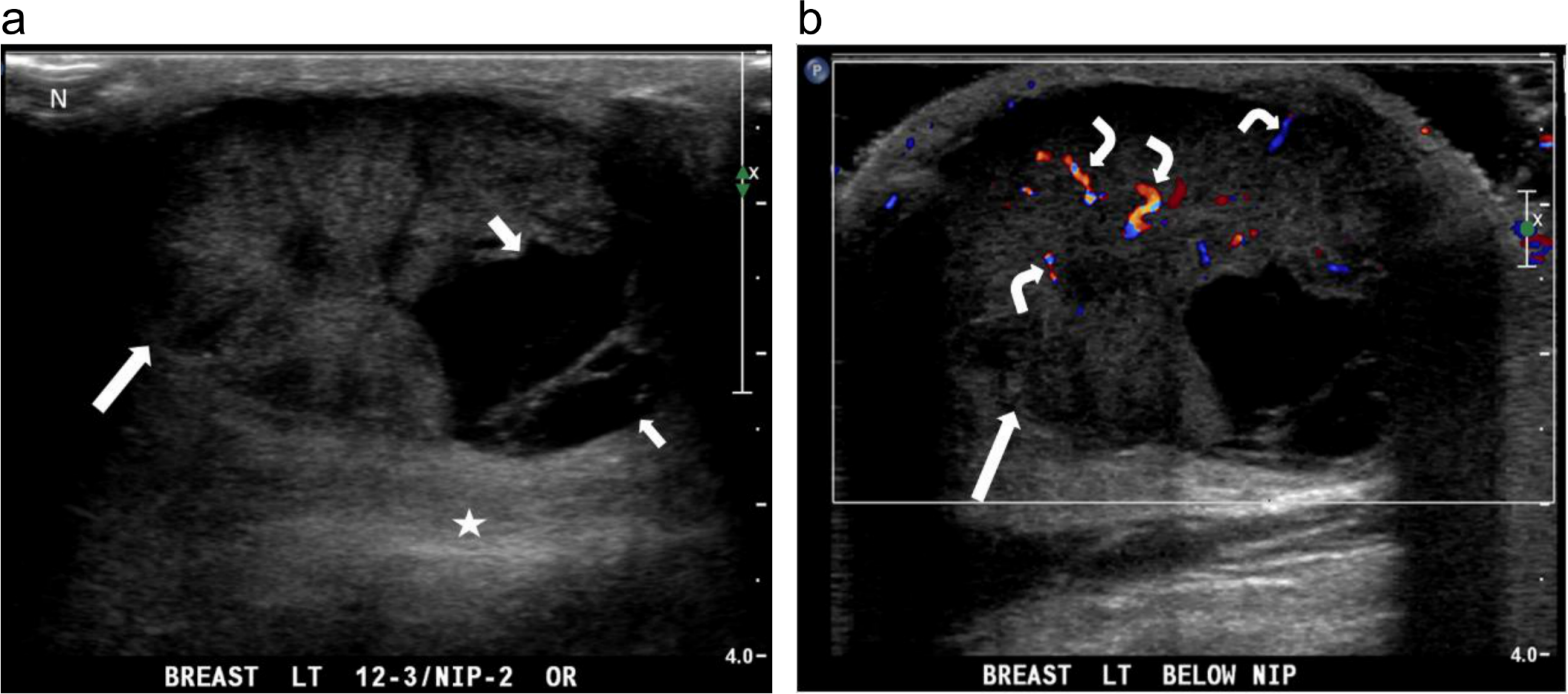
A palpable mass in the left breast of an 84-year-old man. (a) anti-radial ultrasound shows an oval-shaped, circumscribed mass (long arrow) with solid and cystic (small arrows) components. There is posterior acoustic enhancement (star) and a markedly eccentric relationship to the nipple (N). (b) Color Doppler ultrasound shows multiple color pixels and multiple pedicles of blood supply (curve arrows) in the mass, categorized as a rich color flow signal with predominantly internal vascularity. The postoperative pathological diagnosis is invasive ductal carcinoma.
